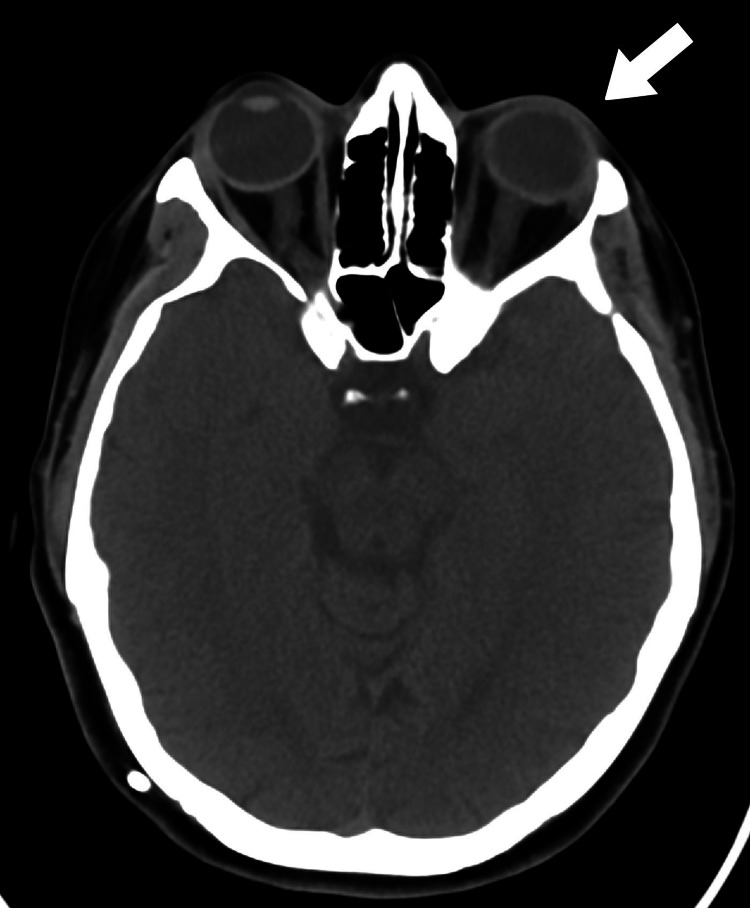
CT axial view The image shows the bilateral enlargement of the optic nerve and perioptic complex with flatting of the optic disc more on the left side with an increase in perioptic cerebrospinal fluid spaces (white arrow) CT: computed tomography
